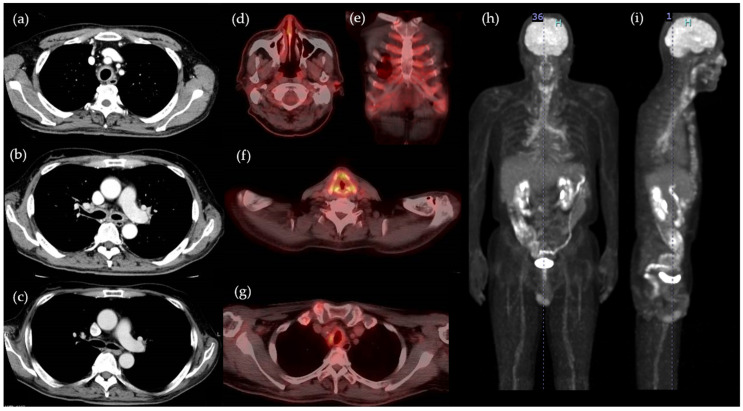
(a,b) Contrast-enhanced CT images of the patient’s tracheal and bronchial walls before the start of treatment. (c) Contrast-enhanced CT images findings of tracheal and bronchial walls on day 36. (d–i) FDG-PET/CT findings of the left auricle, costal cartilage, larynx, and tracheal and bronchial walls before the start of treatment.
